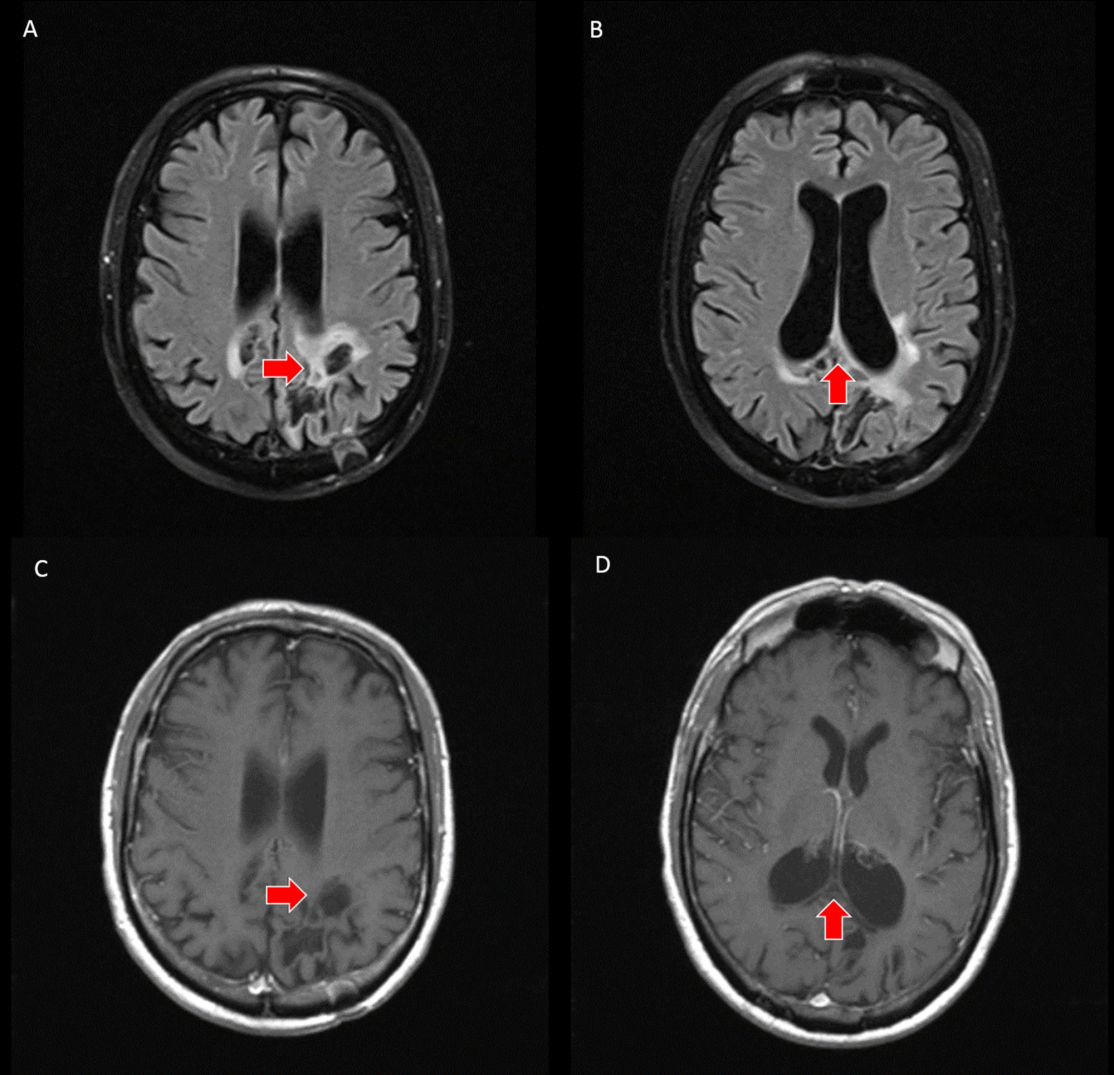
A, B - axial slices from brain MRI following chemotherapy with FLAIR-weighted sequence demonstrates decreased volume of the lesion (red arrows). C, D - axial slices from brain MRI following chemotherapy with T1-weighted pre and post-contrast sequences demonstrate resolution of the previously seen enhancement (red arrows) MRI: magnetic resonance imaging, FLAIR: fluid-attenuated inversion recovery.
